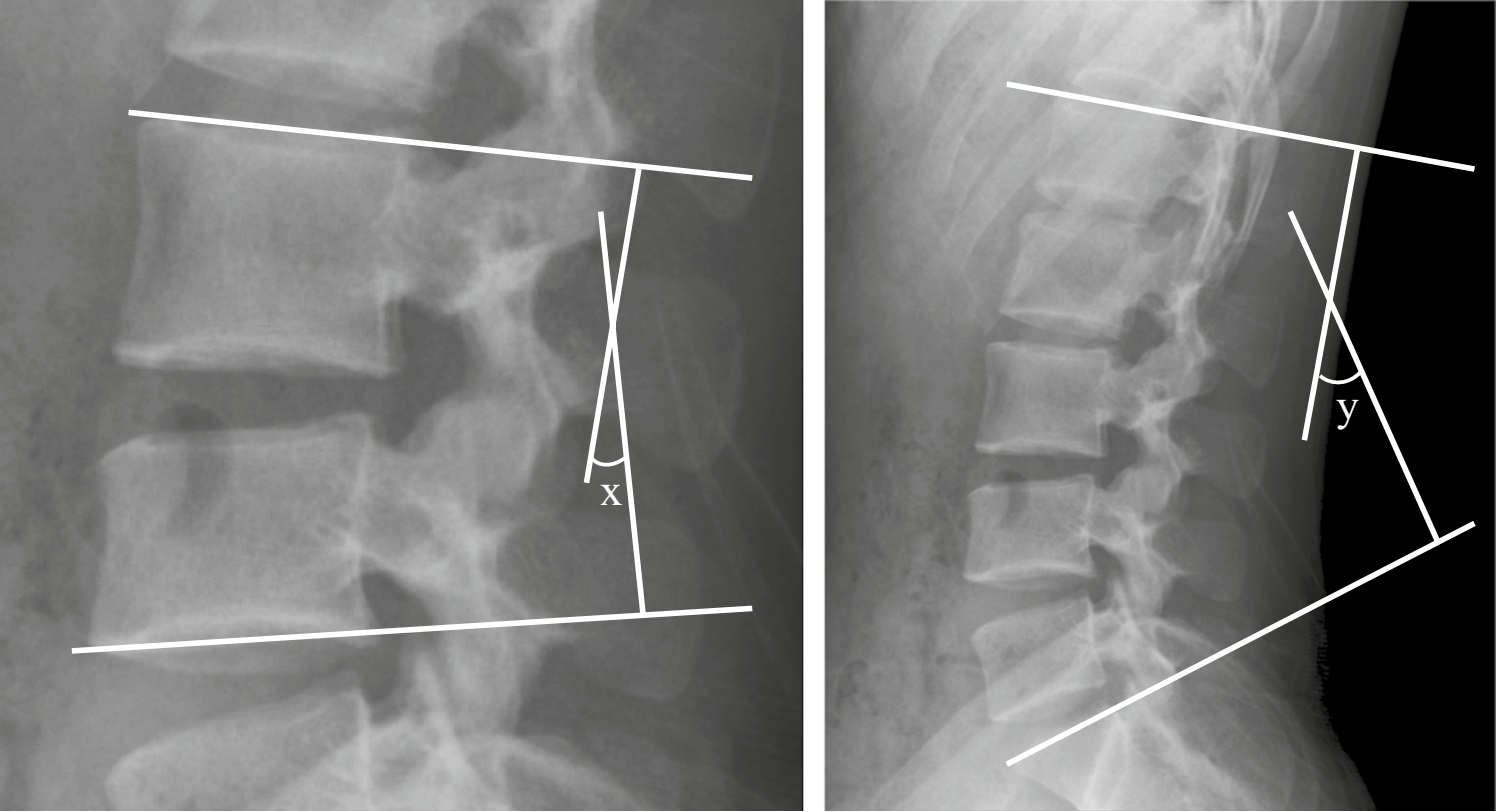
Lateral plain radiograph of the lumbar spine showing measured variables. Segmental angle (x) and total lumbar lordosis (y) were defined at the intersection of lines
